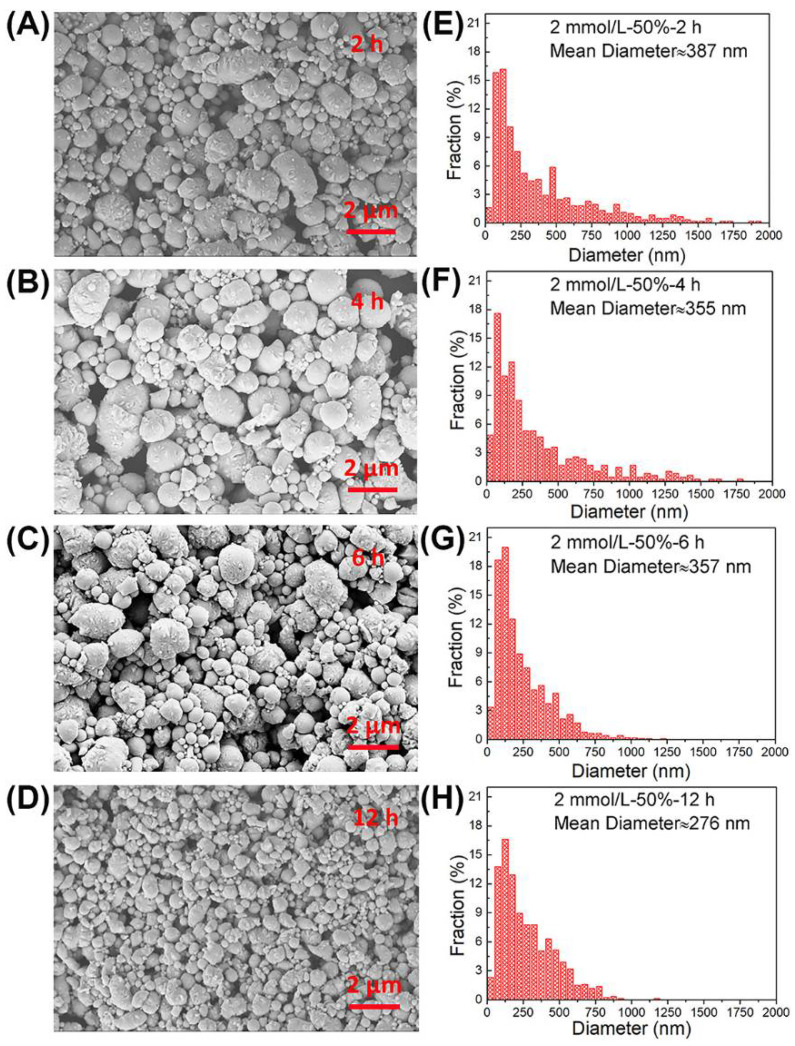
Morphology images and size distributions of eGaIn nanodroplets under different ultrasonic times: (A,E) 2 h (B,F) 4 h (C,G) 6 h and (D,H) 12 h.
